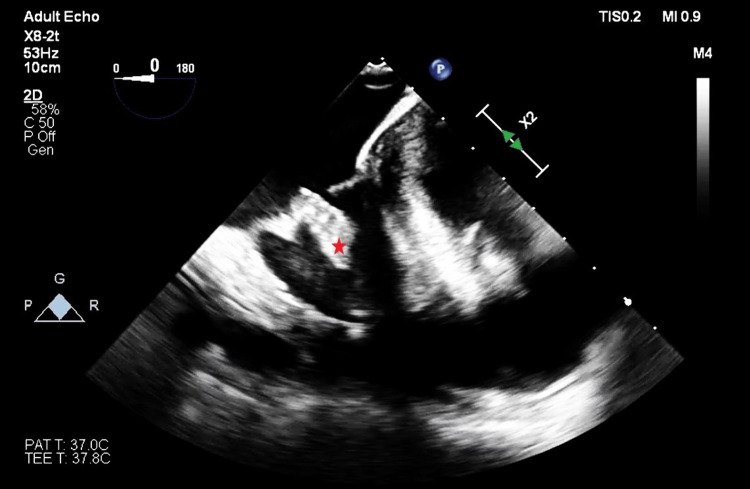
The TEE probe advanced from the mid-esophageal four-chamber view, depicting tricuspid valve mass (red asterisk) likely adhering to the posterior leaflet. TEE:  transesophageal echocardiography
